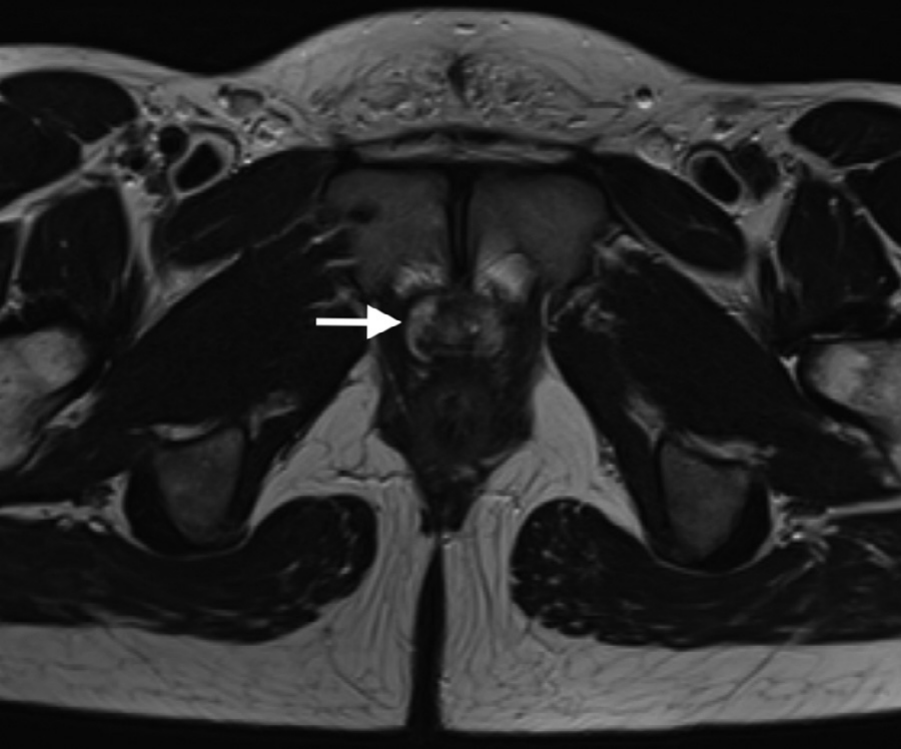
Axial T2-weighted MR image shows periurethral prostate tissue (arrow) with evidence of a hypointense transitional zone and a hyperintense peripheral zone.
